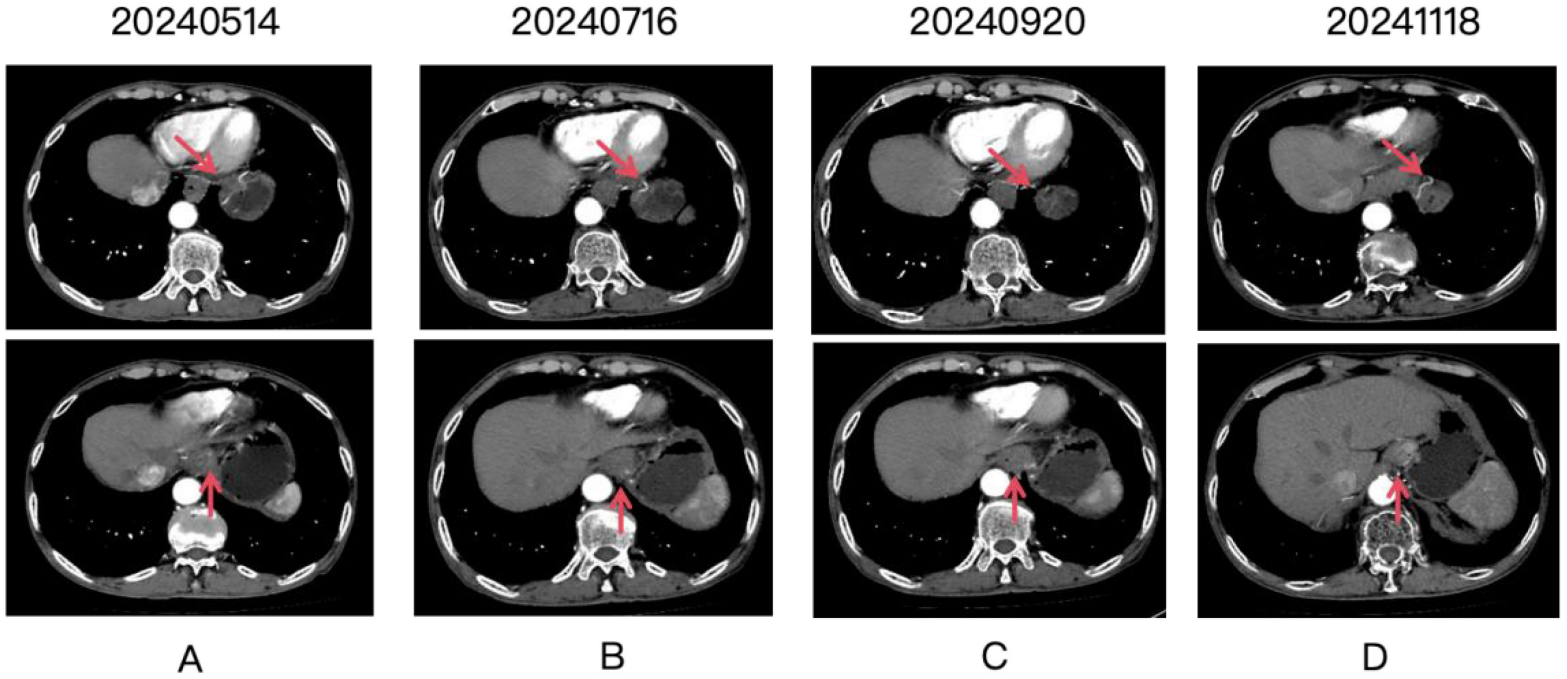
Four panels labeled (A-D) show CT scan images over time, with dates 20240514, 20240716, 20240920, and 20241118. Each panel contains two images. Red arrows point to the gastric lesion, which shows a partial response after treatment.
